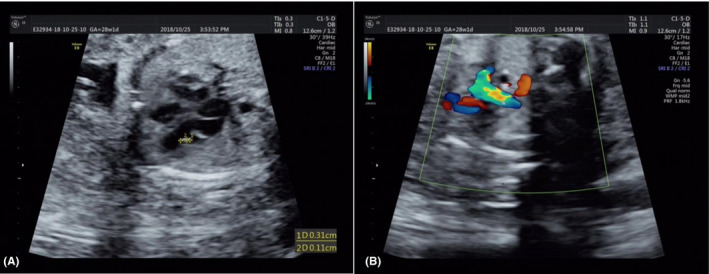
Prenatal ultrasound findings at 28 wk of gestation: A, Intracardiac echogenic focus in the left ventricle; B, Aberrant right subclavian artery
